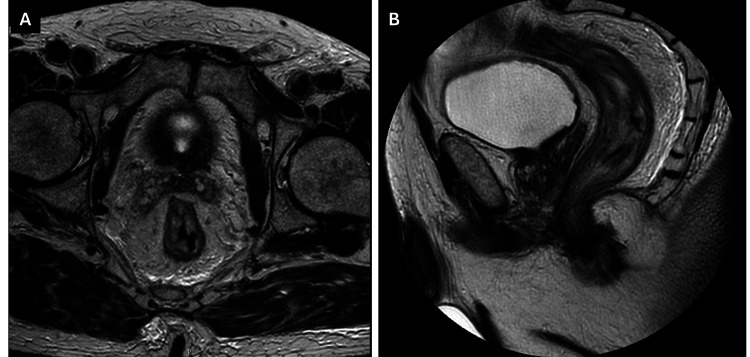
Post-neoadjuvant therapy T2-weighted magnetic resonance images demonstrating complete remission of the rectal tumor. A - axial; B - sagittal
